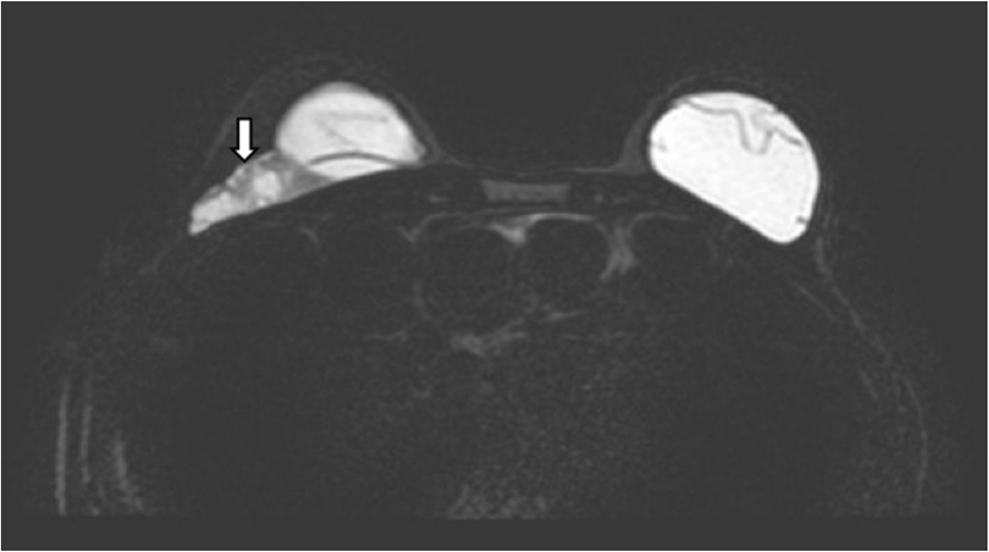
Extracapsular rupture of the right silicone gel-filled implant. Silicone-only axial MR image shows extracapsular rupture of the right silicone gel-filled implant (arrow)
